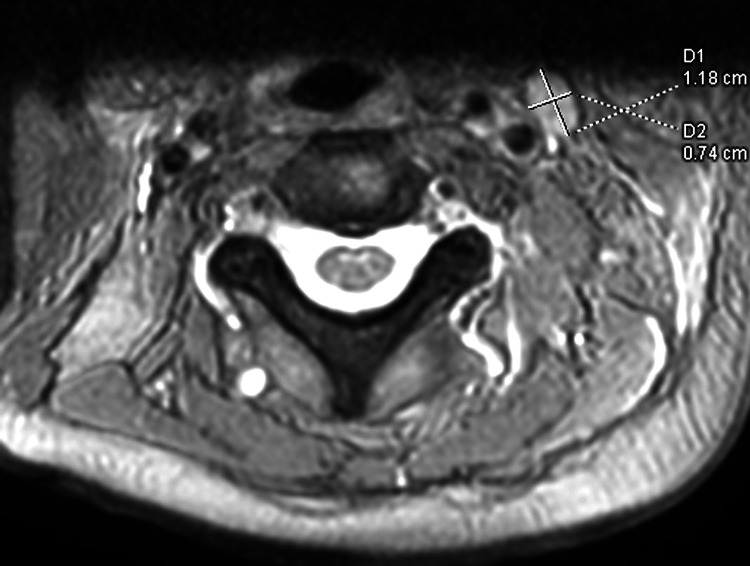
Coronal view of the cervical MRI. Few prominent left cervical lymph nodes (up 1.18 cm x 0.74 cm) are identified, which can represent metastatic or reactive node.
